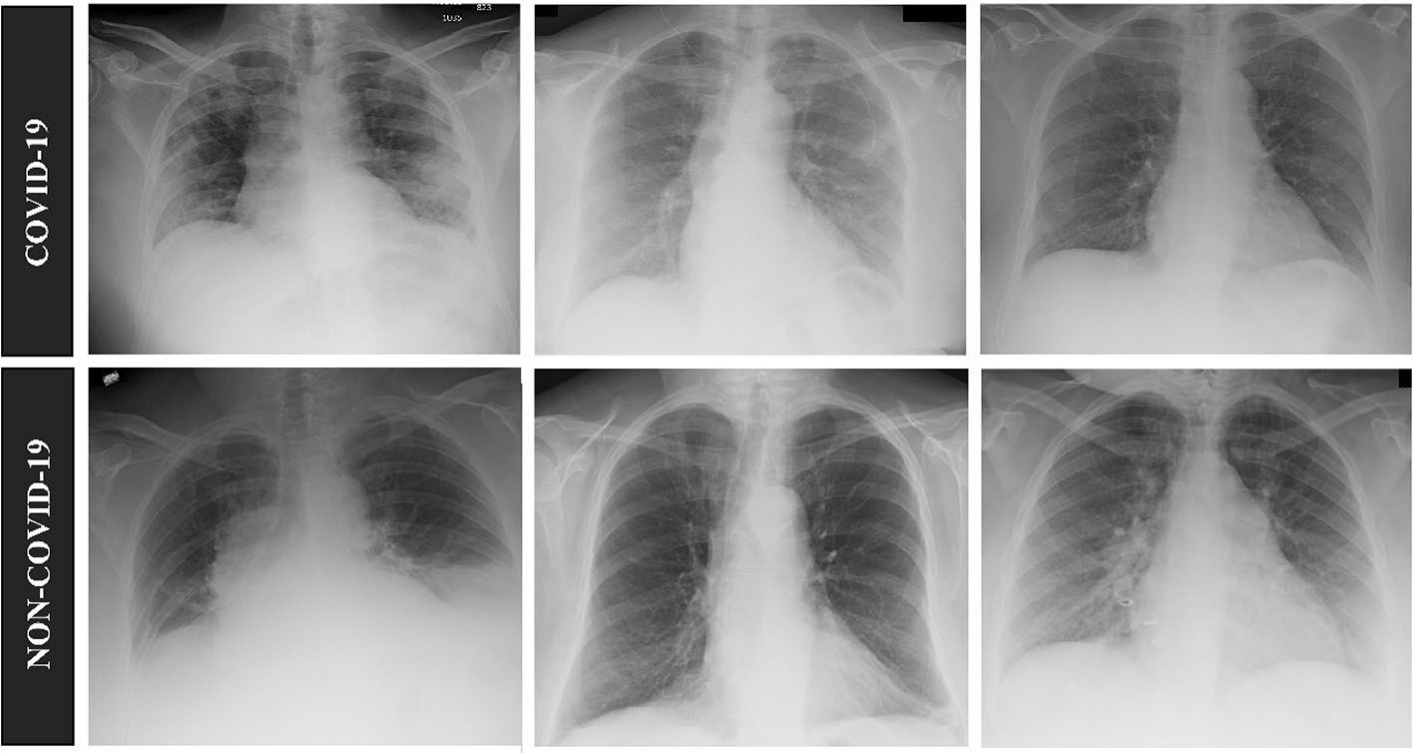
Examples of COVID-19 and non-COVID-19 chest X-ray images.
